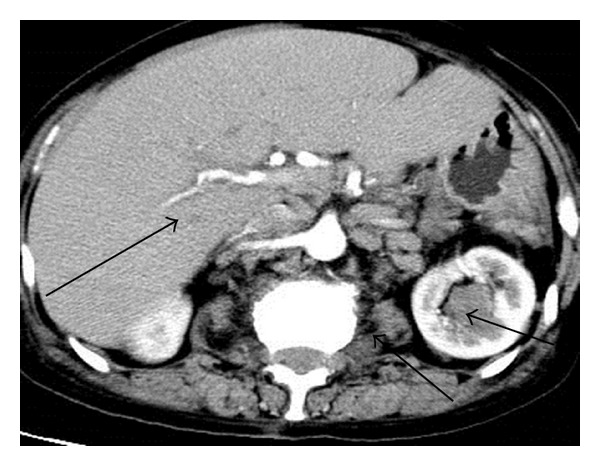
CT result shows multiple lymph nodes in the peritoneal cavity and retroperitoneal area. An enlarged liver and soft tissue masses were observed in the left kidney. On November 13, 2009, CT scan was performed, and the left arrow indicated enlarged liver with homogenous density, the middle arrow suggested enlarged lymph nodes in the abdomen, and the right arrow represented a soft tissue mass in the left kidney.
